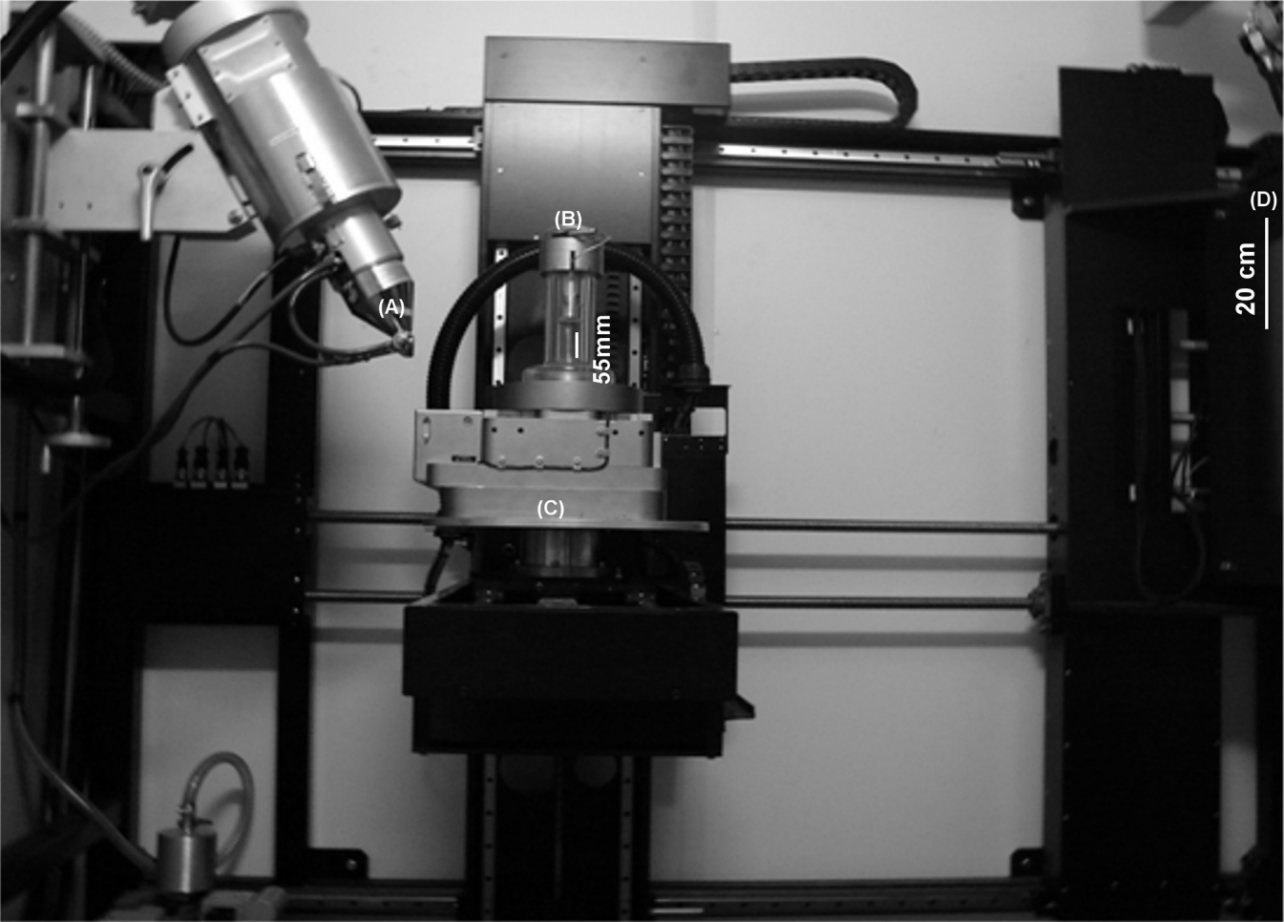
Nikon Custom Bay 320Kv X-ray micro-tomography system. The figure shows (A) the source, (B) the mechanical rig with the bone mounted for testing inside the perspex tube, (C) the load assembly and (D) the detector.
